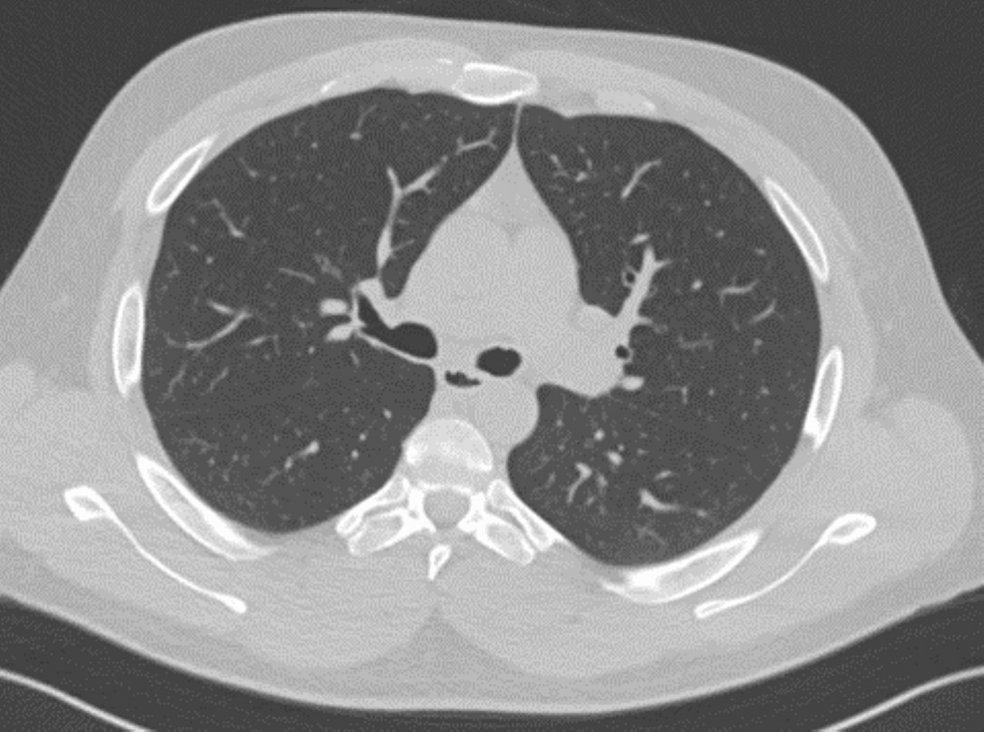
Repeat CT of the chest during four-week follow-up showing the complete resolution of pneumomediastinum and ground glass opacities.
